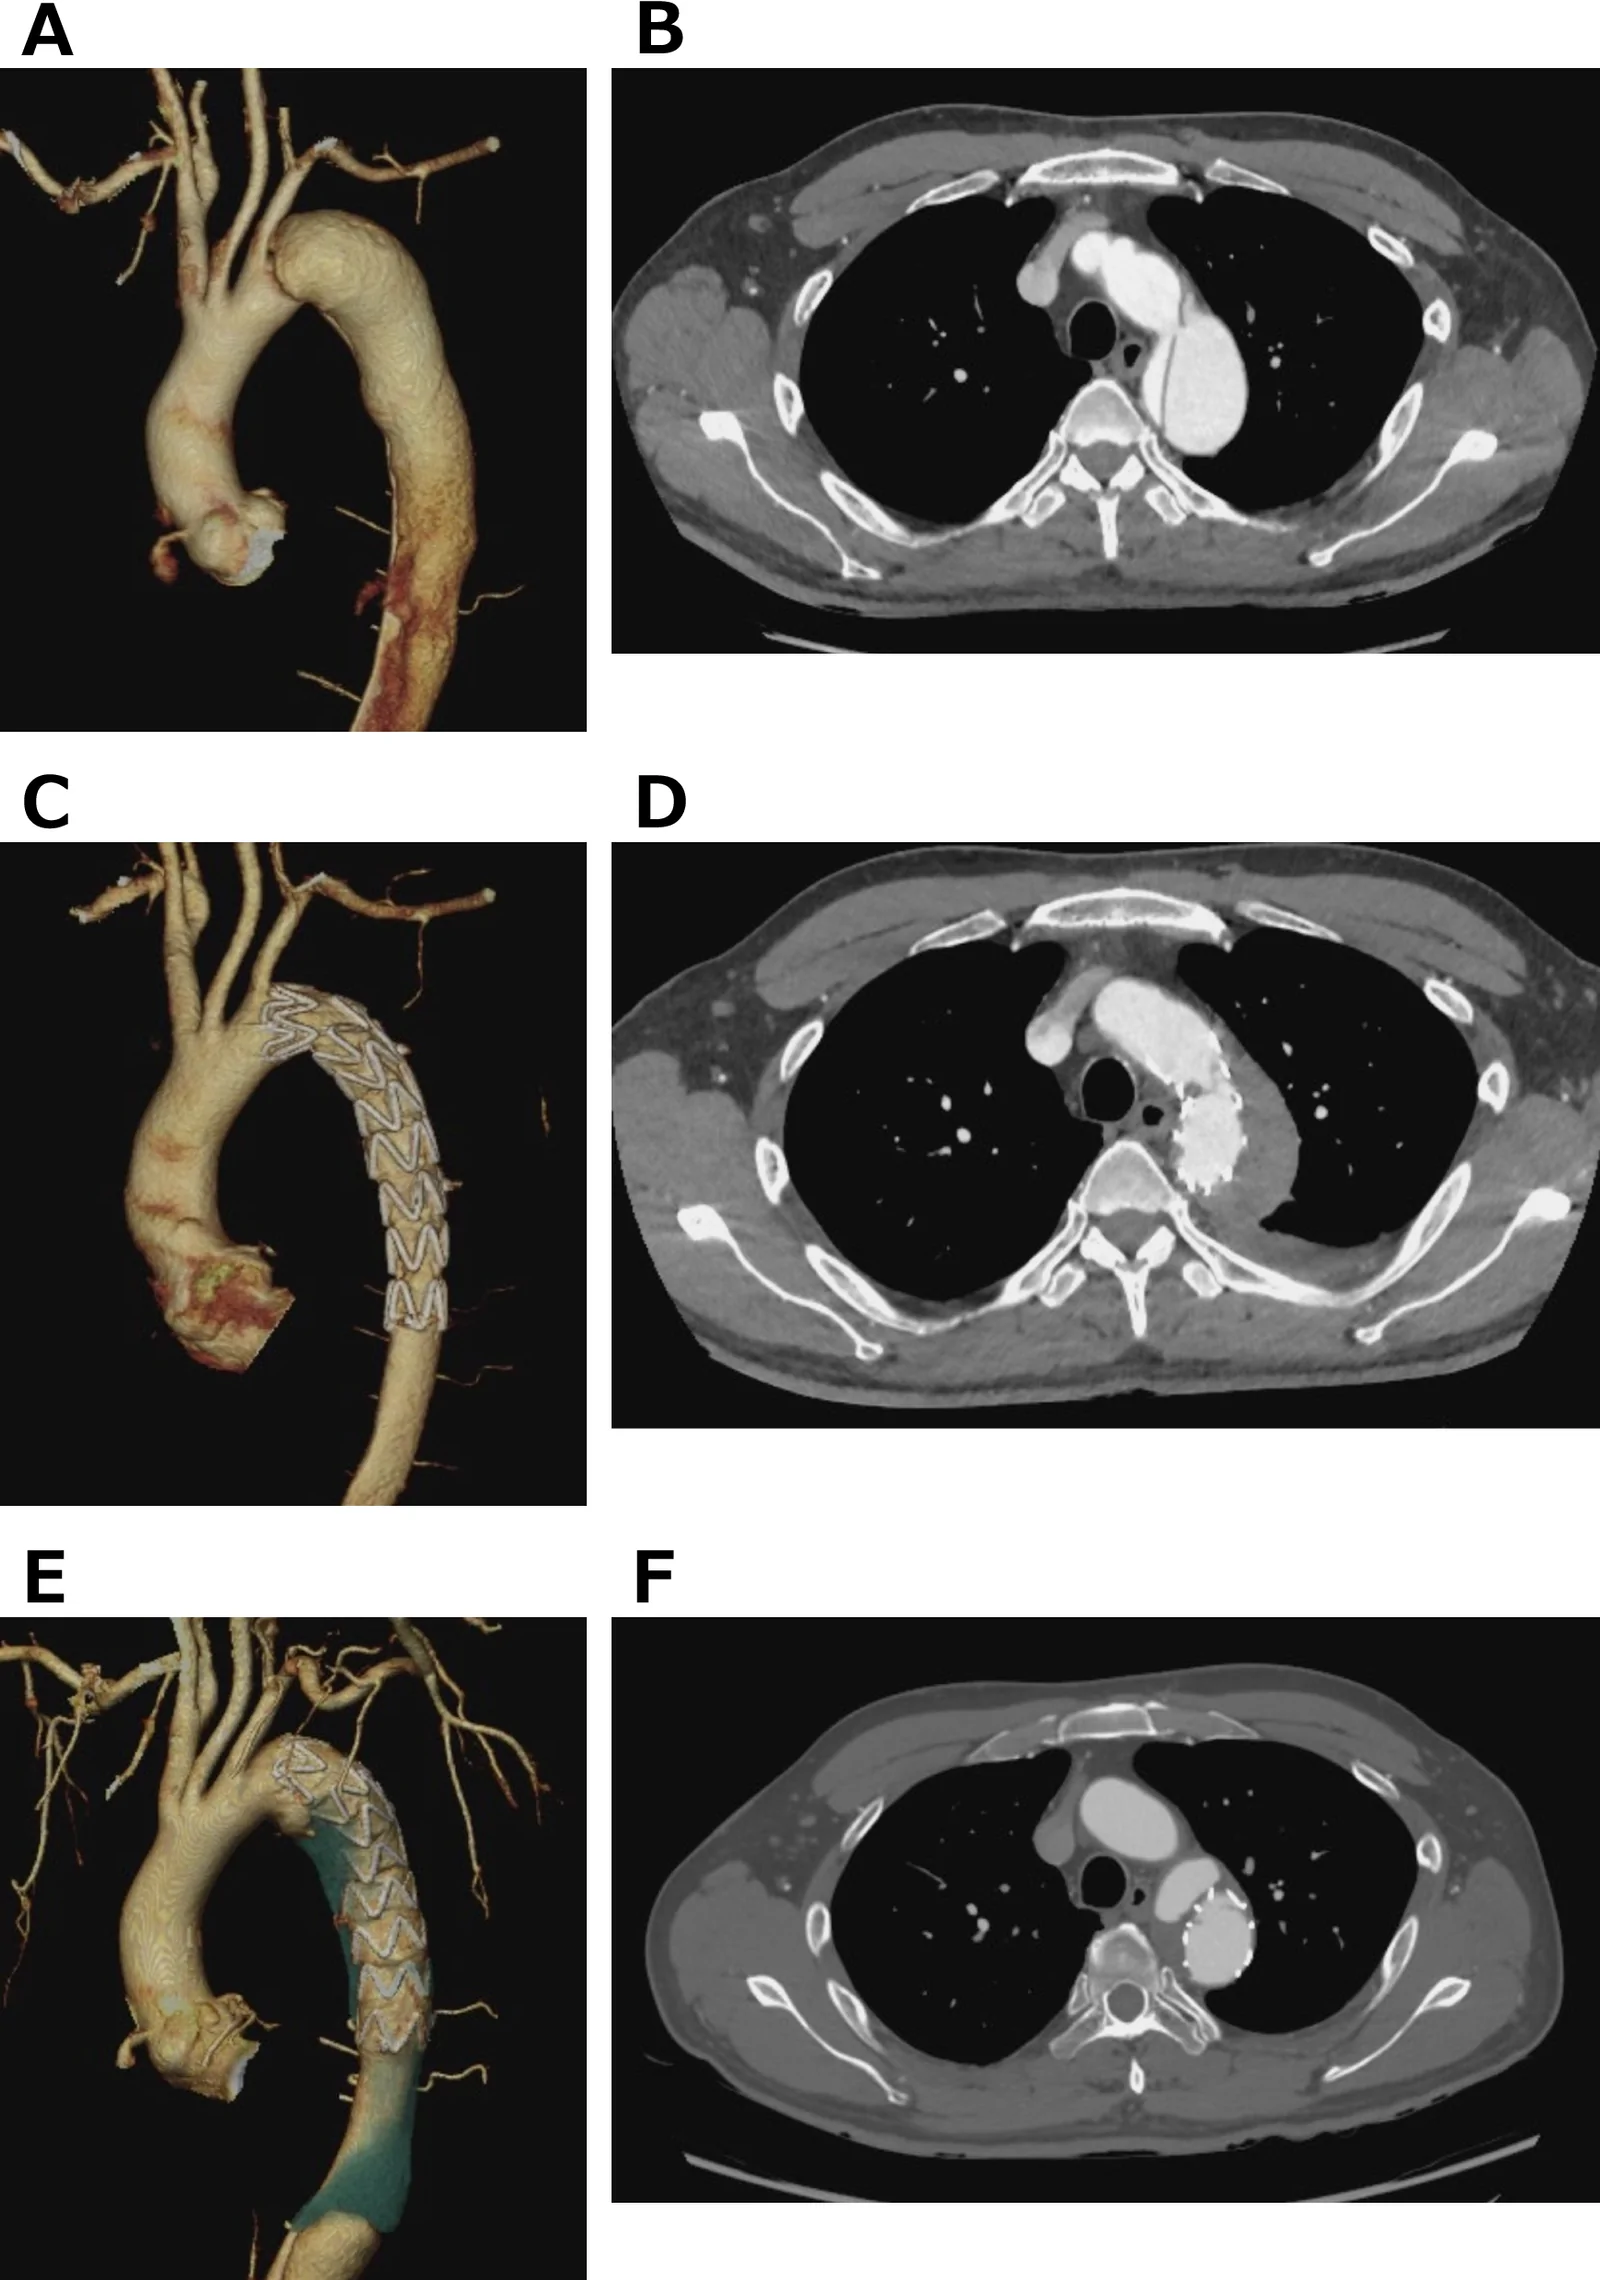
Serial CT findings during the clinical course. A 3D CT after the onset of acute type B aortic dissection and prior to preemptive TEVAR. B Axial contrast-enhanced CT after the onset of acute type B aortic dissection and prior to preemptive TEVAR. C Postoperative 3D CT after preemptive TEVAR. D Postoperative contrast-enhanced CT after preemptive TEVAR. E Preoperative 3D CT before PM-F/iBEVAR. F Preoperative contrast-enhanced CT before PM-F/iBEVAR. CT: computed tomography; 3D: 3-dimensional; TEVAR: thoracic endovascular aortic repair; PM-F/iBEVAR: physician-modified fenestrated/inner-branched endovascular aortic repair
